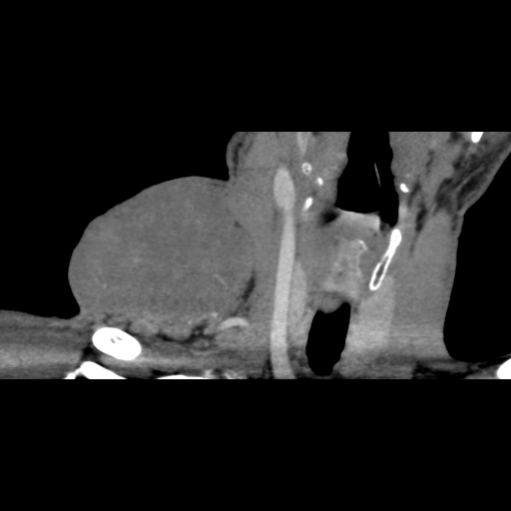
Transversal CT reconstruction
